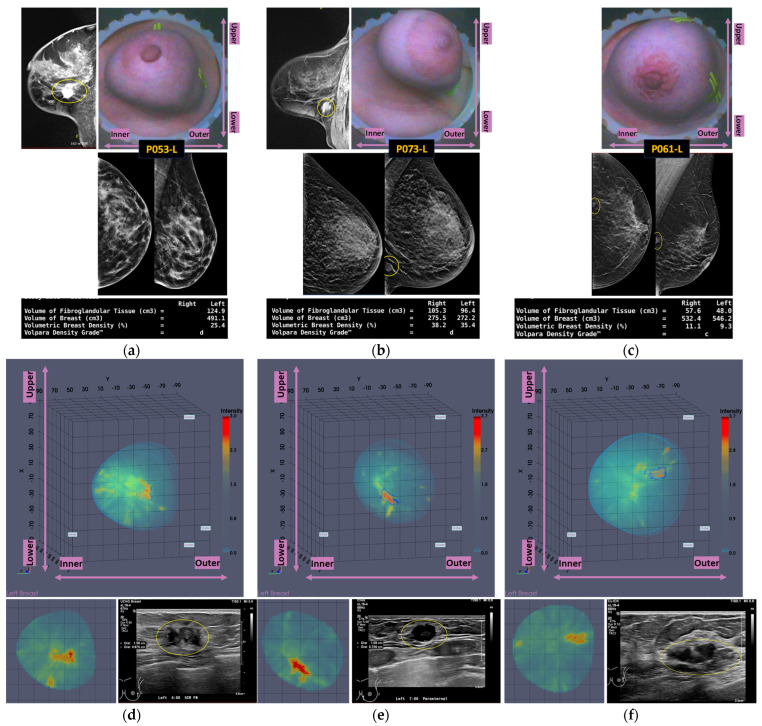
Reference and Wavelia MWBI images for the 3 confusing cases in the malignant-to-benign lesion separability analysis space: (a,d) P053-L, (b,e) P073-L, and (c,f) P061-L. Top row: endoscopic camera view of each breast after positioning in the MWBI scanner—depicted view aligned with the orientation of the MWBI 3D image’s Default View; CC and MLO mammographic views of the breast; Volpara Lab—mammographic computational outputs (Volumetric Breast Density (VBD) and Breast Volume); and sagittal slice highlighting the lesion in the breast MRI scan (if available). Bottom Row: Wavelia MWBI 3D image’s Default View, with the extracted ROI delineated and superimposed in blue; coronal XY slice of the MWBI image cut at the centroid of the clinically relevant ROI in the MWBI image; and snapshot of the ultrasound scan of the breast provided as reference data for the same lesion in this study.
